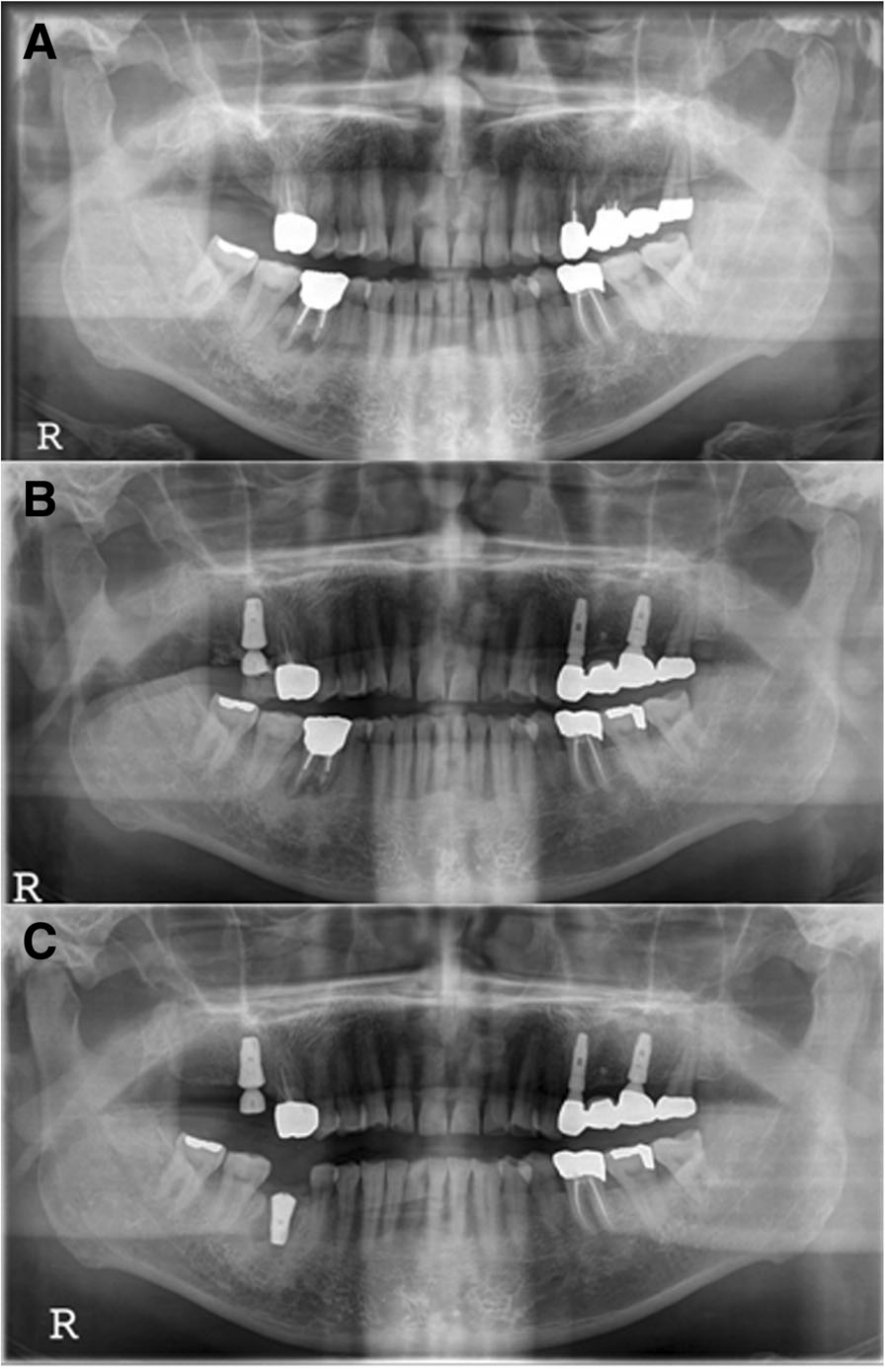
M3, panoramic radiograph, #46i surgery a initial panoramic radiograph, b pre-operative panoramic radiograph, c postoperative panoramic radiograph
